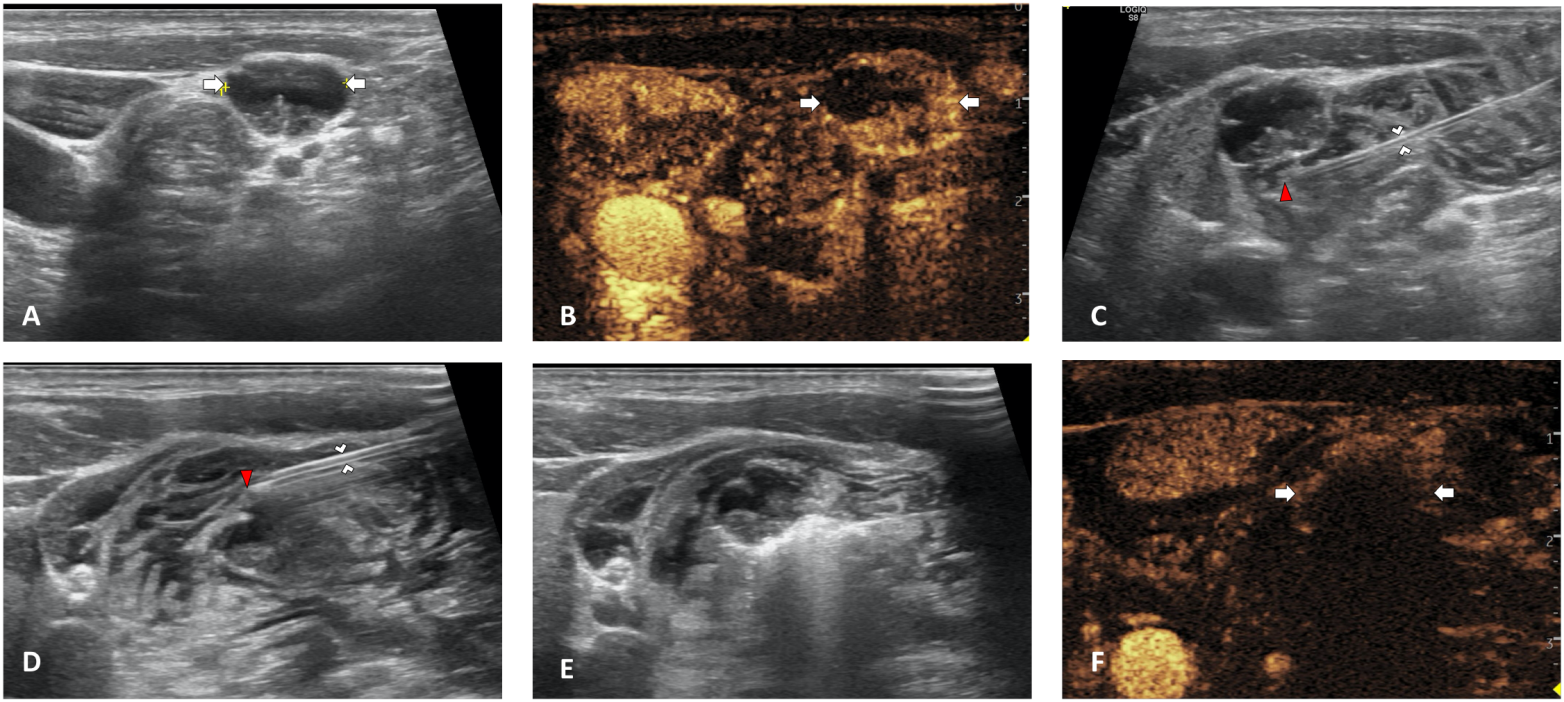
Ultrasound images of traditional hydrodissection. (A) Pre-MWA, B-mode ultrasonography (US) showed hypoechoic LN (white arrow). (B) Pre-MWA, Contrast-enhanced ultrasound (CEUS) showed inhomogeneous enhanced LN (white arrow). (C) Traditional hydrodissection before ablation. The needle tip (red arrowhead) was placed in the posterior fascial spaces outside the LNM capsule and forming a narrow anechoic isolating band outside the LNM lesion in the posterior space. (D) Traditional hydrodissection before ablation. The needle tip (red arrowhead) was placed in the anterior fascial spaces outside the LNM capsule and forming a narrow anechoic isolating band outside the LNM lesion in the anterior space. (E) During ablation, the thickness of isolating band was maintained through continuous injection of NS. (F) Post-MWA, CEUS showed no enhancement in LN (white arrows).
